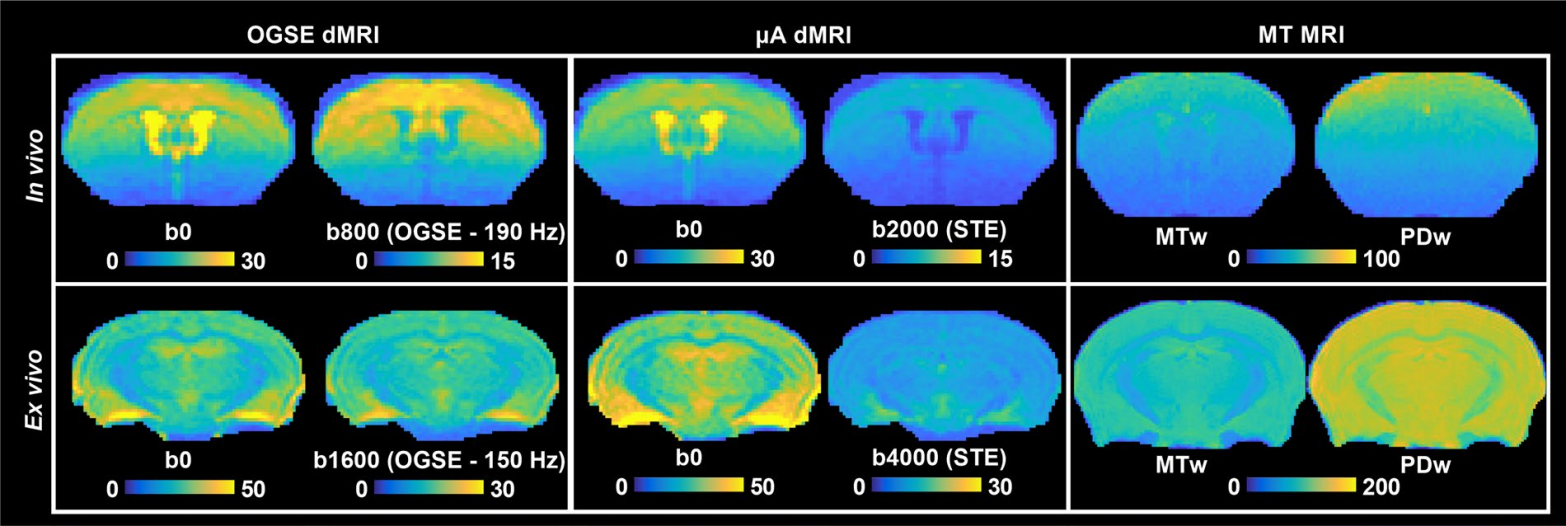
SNR maps of *in vivo* and *ex vivo* images. SNR maps for a single b = 0 s/mm^2^ image are shown for all dMRI protocols, and SNR maps for the powder average of the highest b-values are shown for all protocols (b = 800 s/mm^2^ for OGSE-190 Hz (*in vivo*), b = 2000 s/mm^2^ for μA-STE (*in vivo*), b = 1600 s/mm^2^ for OGSE-150 Hz (*ex vivo*), and b = 4000 s/mm^2^ for μA-STE (*ex vivo*)). SNR maps for MTw and PDw scans are shown for MT MRI. *Adapted from Rahman et al*.^2^.
